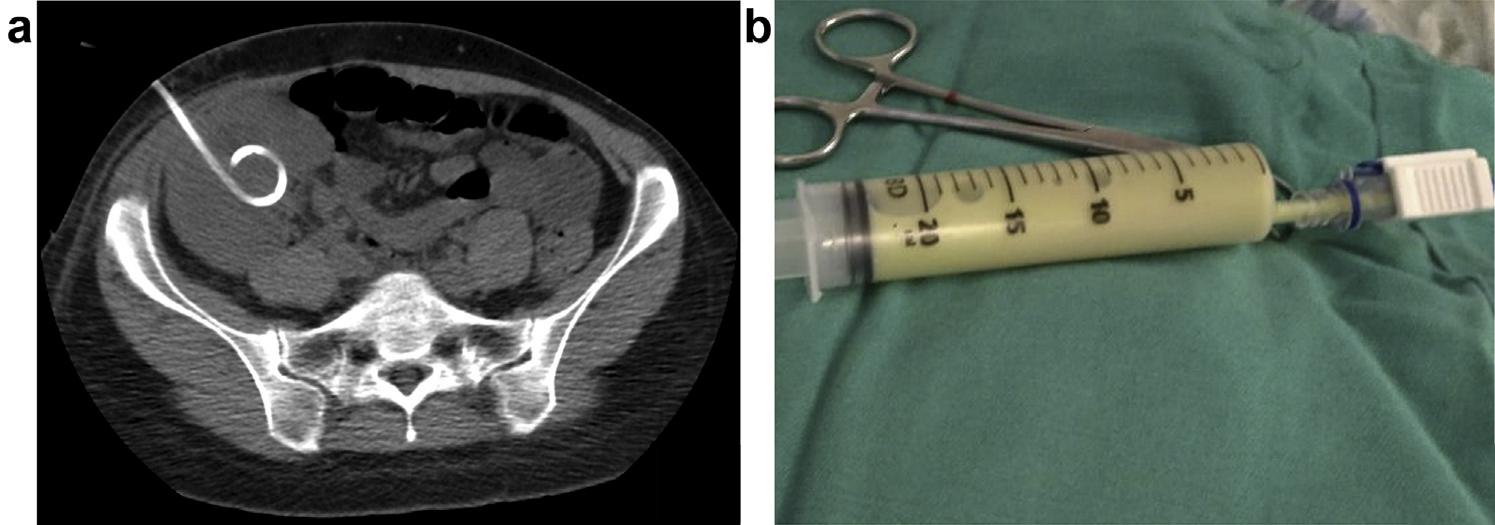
Percutaneous computed tomography−guided drainage of (a) the simple cyst with (b) purulent secretion.
